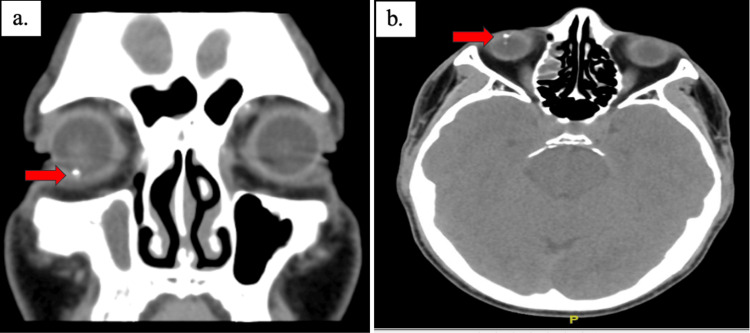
Coronal view of plain computed tomography of the orbit showing a hyperdense focus seen at the inferior aspect (a); axial view showing two hyperdense foci at the inferior aspect of the posterior segment, suggestive of intraocular foreign bodies (IOFB) in the right globe (b).
